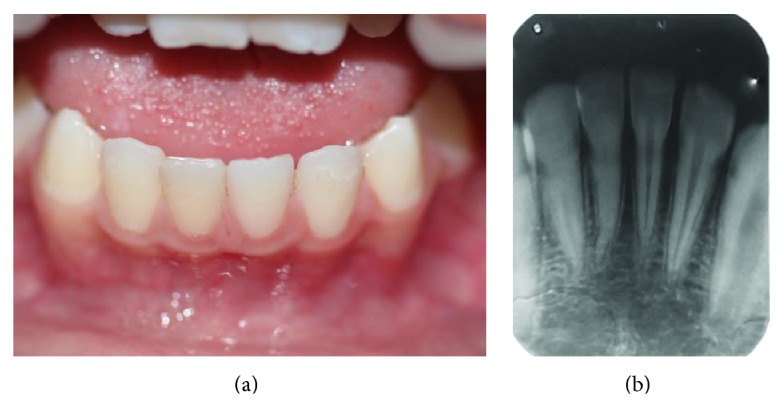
(a) Five-year clinical follow-up. (b) Radiographic image after 5 years of the trauma. Full closure of the apexes and marked obliteration of the root canal lumens.
